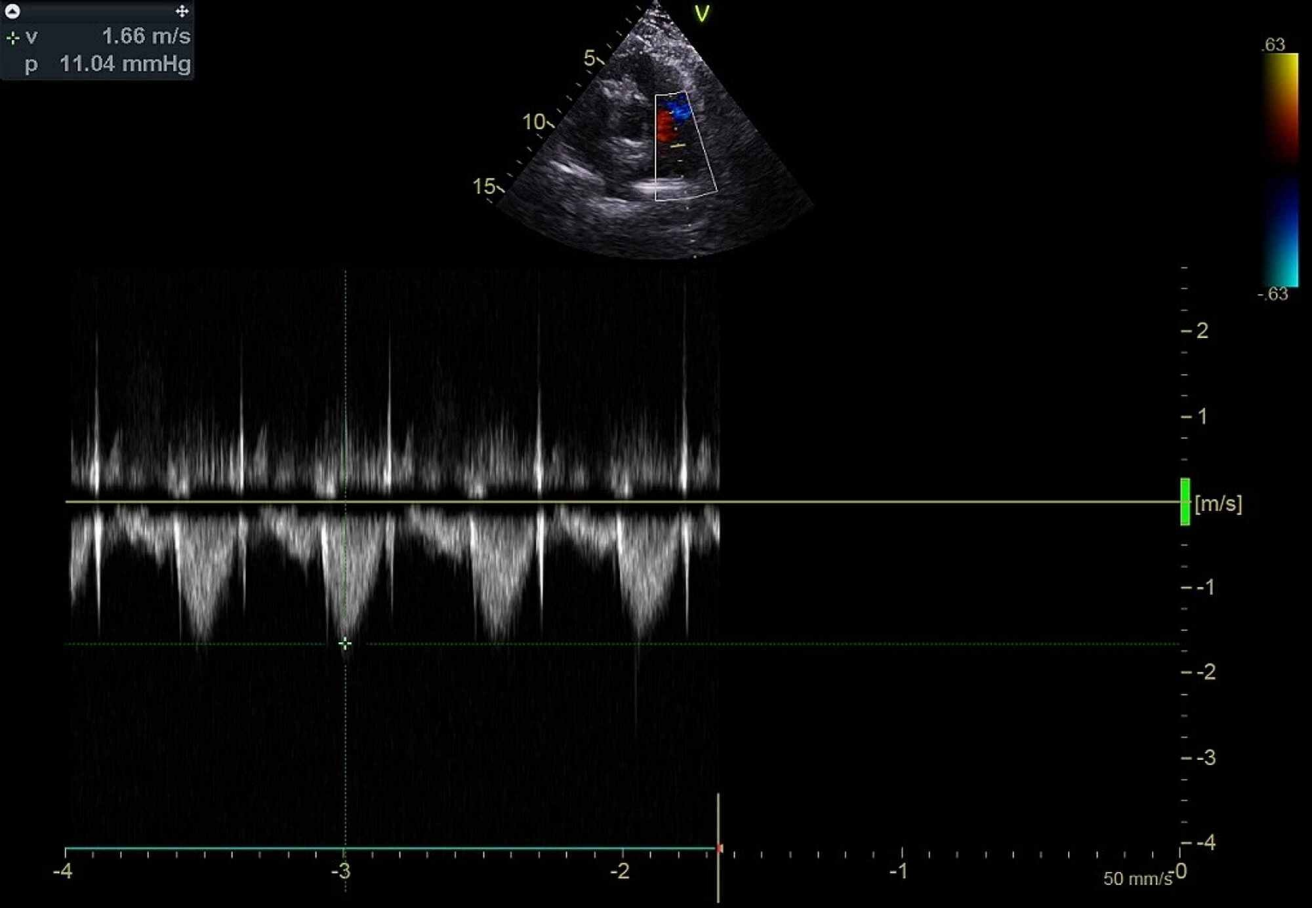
Continuous wave Doppler signals from the pulmonary valve showing normal flow velocity
